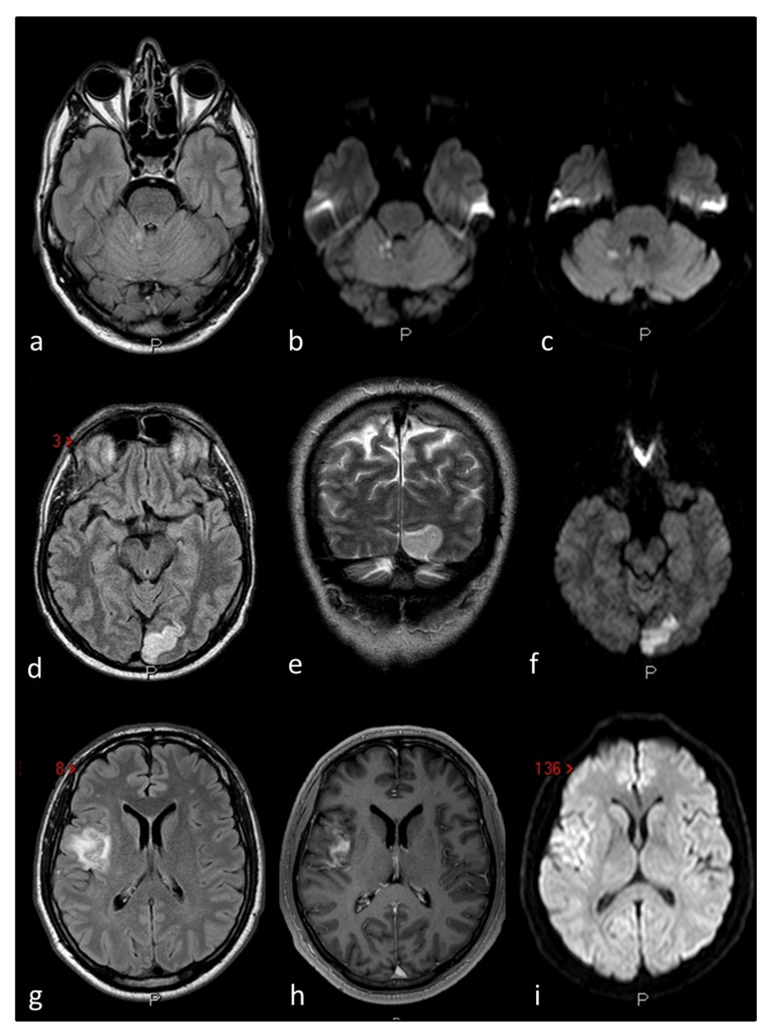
The figure outlines three examples of cryptogenic embolism in patients with PFO as imaged in Magnetic Resonance Imaging (MRI) of the brain: Patient 1 (a–c) multiple right cerebellar recent ischemic lesions on axial FLAIR (a) and DWI (b,c) MRI sequences. Patient 2 (d–f) left occipital ischemic stroke in a patient with migraine with aura and PFO on axial FLAIR (d) coronal T2W (e) and DWI MRI sequences. Patient 3 (g–i) right temporo-parietal ischemic stroke on axial FLAIR (g) contrast-enhanced axial T1W (h) and DWI (i) MRI sequences.
